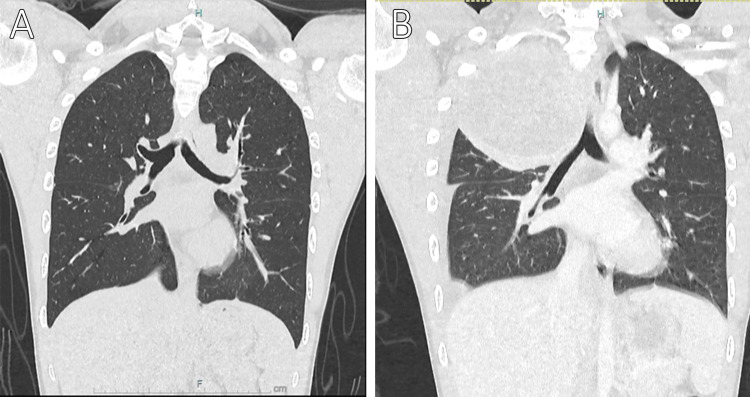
Serial contrast-enhanced computed tomography (CECT) from first encounter (A) and three weeks later (B) showing now a large heterogeneously enhancing mass occupying the right upper lobe, compressing onto the right upper lobe bronchus.
